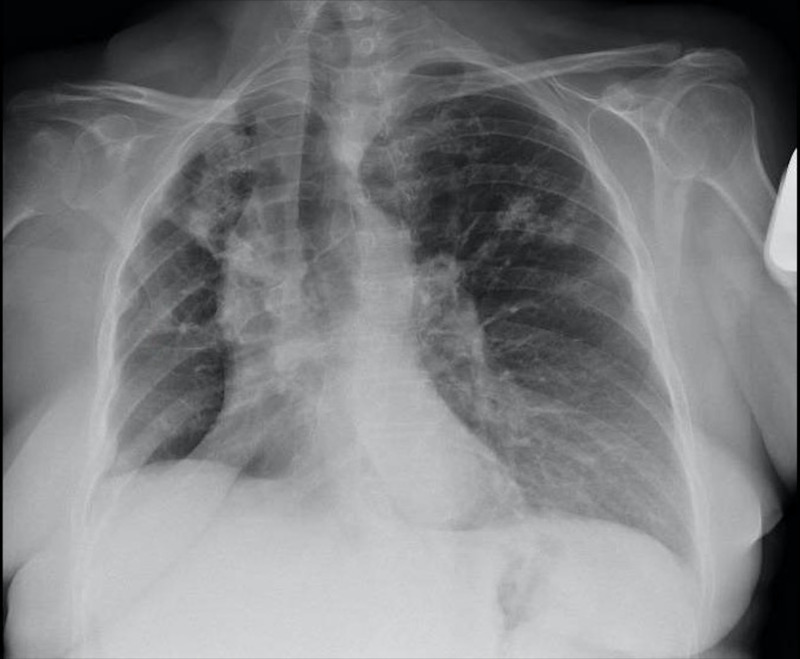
Chest X-ray image showing enlarged hilar shadow and interstitial infiltrates in the right upper and middle lobes
